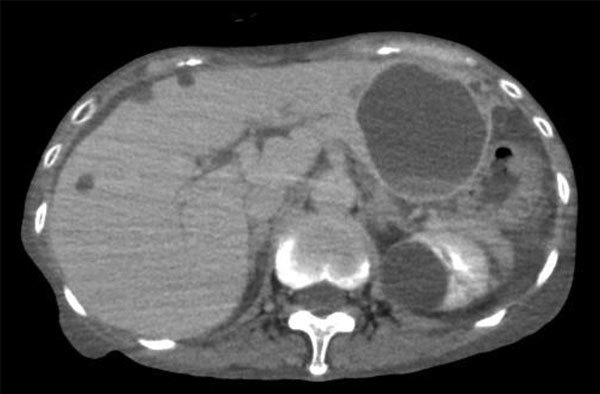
Enhanced computed tomography image showing a large hepatic cyst (63 mm in diameter) on the left liver lobe in a patient with *Helicobacter cinaedi* infection on the day of hospital admission, Tokyo, Japan, July 2017.
